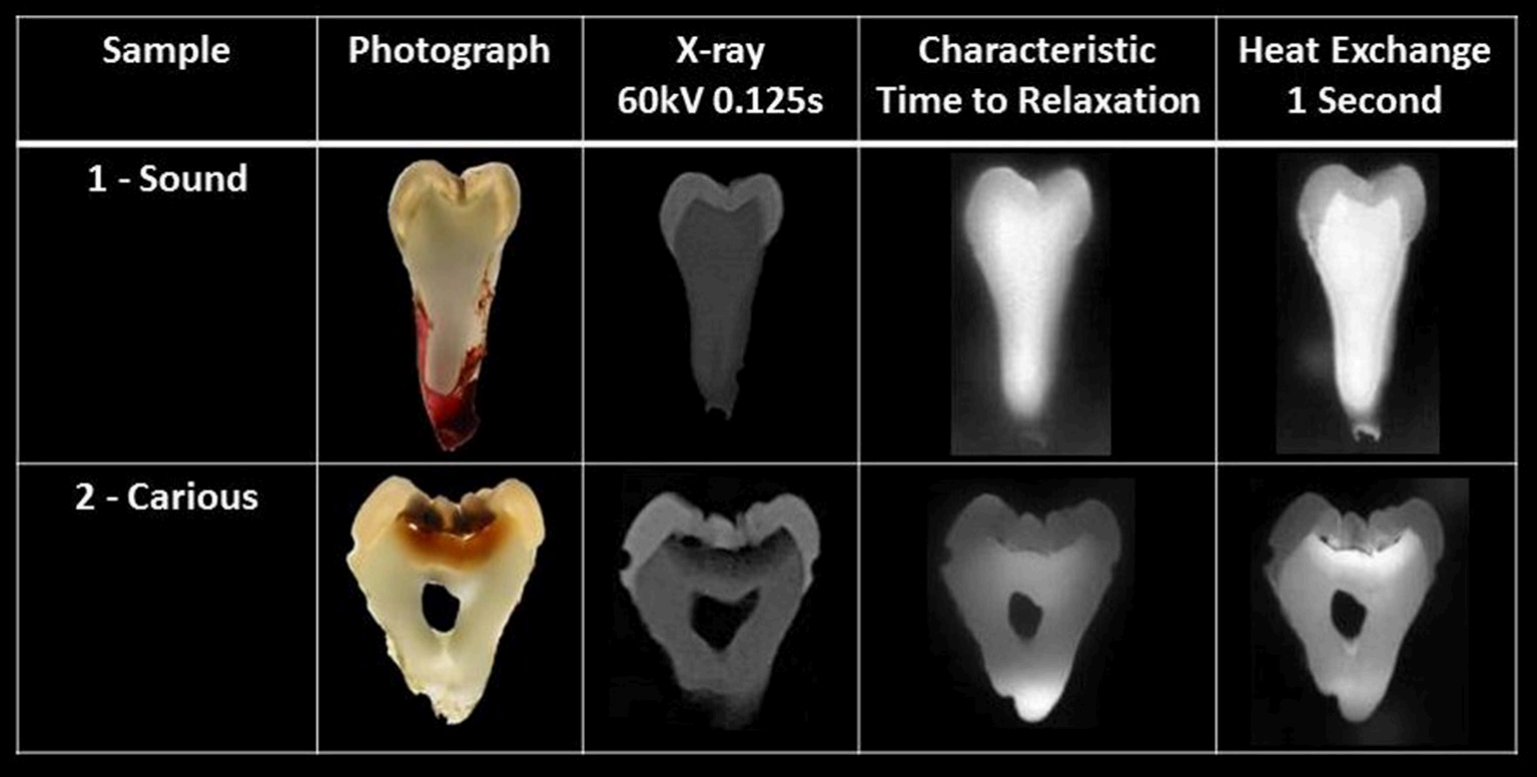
Sample 1- Sound tooth-slice and Sample 2 - Carious tooth-slice. Initially, a photograph is shown, followed by an X-ray, then the characteristic-time-to-relaxation thermal map and, finally, the heat-exchange thermal map depicting enamel, dentin and carious areas of enamel and dentin.
